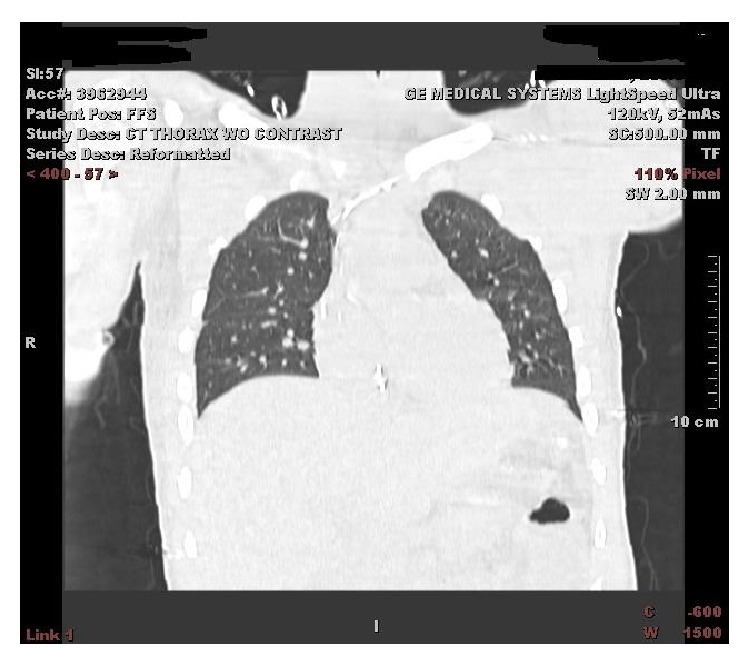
Confirmatory CT of radiopaque catheter, 10 cm, tubing extending from retrohepatic IVC through the right atrium into the low SVC.
